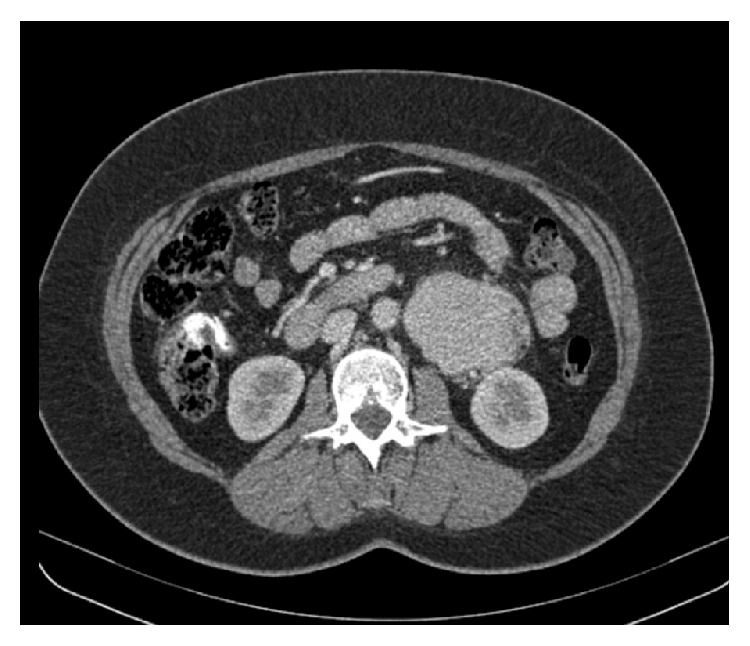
Preoperative abdominal computed tomography (CT) scan depicting a voluminous left pararenal mass.
